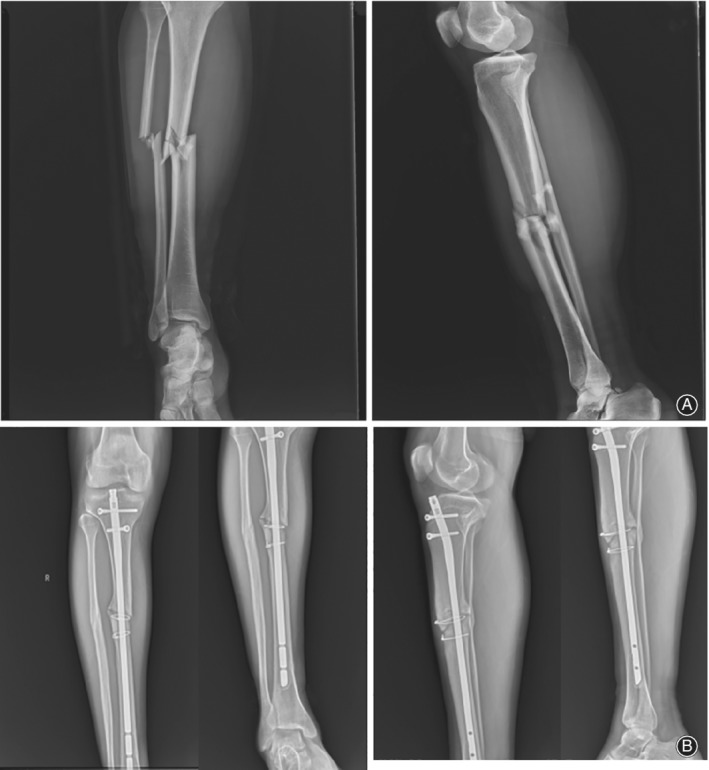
(A) The lateral radiograph of the tibiofibula shows the comminuted fracture of the right tibiofibular; the fracture was obviously displaced. (B) Three years after surgery, the lateral radiograph of the tibiofibula shows the nonunion of the right tibia; the fracture line is clear and sclerotic bone formation can be seen at the fracture end.
